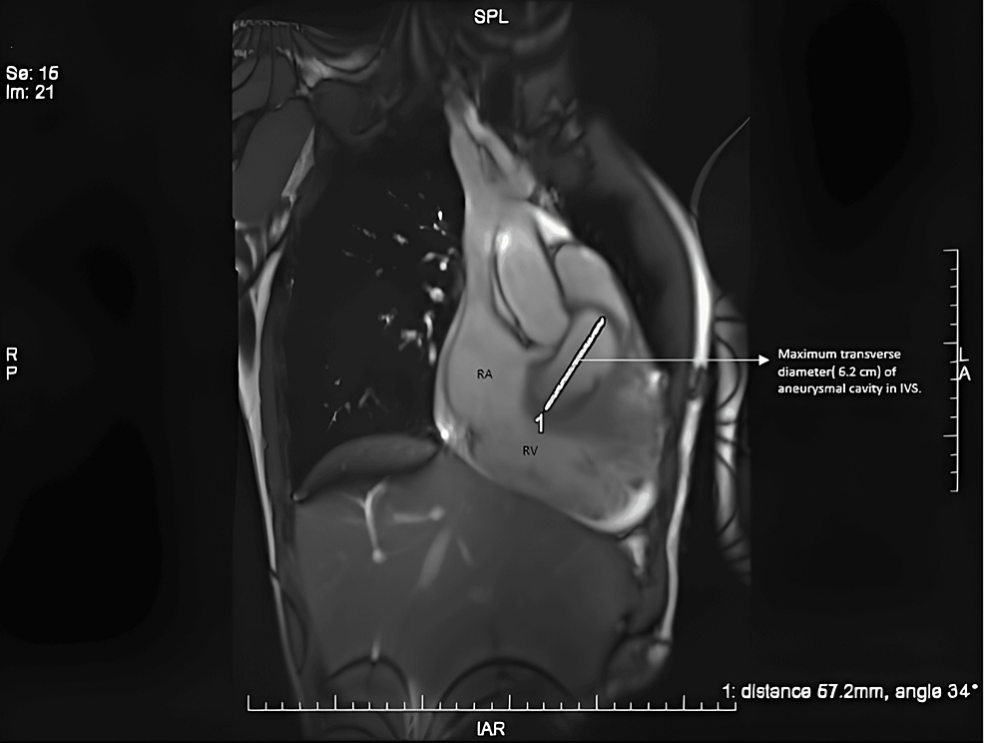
Cardiac MRI depicts the maximum dimension (57.2 mm) of the aneurysmal cavity within the interventricular septum (IVS).
